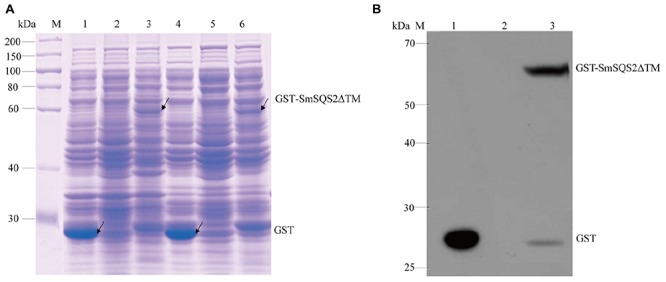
Characterization of recombinant protein. (A) SDS-PAGE analysis of recombinant *SmSQS2* protein expressed in *Escherichia coli*. M: protein marker. Lane 1-3( total cell extract), 1: *pGEX-4T-1* bacteria of after induce; 2: *pGEX-4T-1-SmSQS2*Δ*TM* bacteria of before induce;3: *pGEX-4T-1-SmSQS2*Δ*TM* bacteria of after induce; Lanes 4–6(supernatant of the cell lysate treated),4: *pGEX-4T-1* bacteria of after induce; 5: *pGEX-4T-1-SmSQS2*Δ*TM* bacteria of before induce; 6: *pGEX-4T-1-SmSQS2*Δ*TM* bacteria of after induce. (B) Western Blot assay of the recombinant *SmSQS2* protein expressed in *E. coli*. M: protein marker; 1: supernatant of the *pGEX-4T-1* ultrasound crushing after induce; 2: supernatant of the *pGEX-4T-1-SmSQS2*Δ*TM* ultrasound crushing before induce; 3: supernatant of the *pGEX-4T-1-SmSQS2*Δ*TM* ultrasound crushing after induce.
